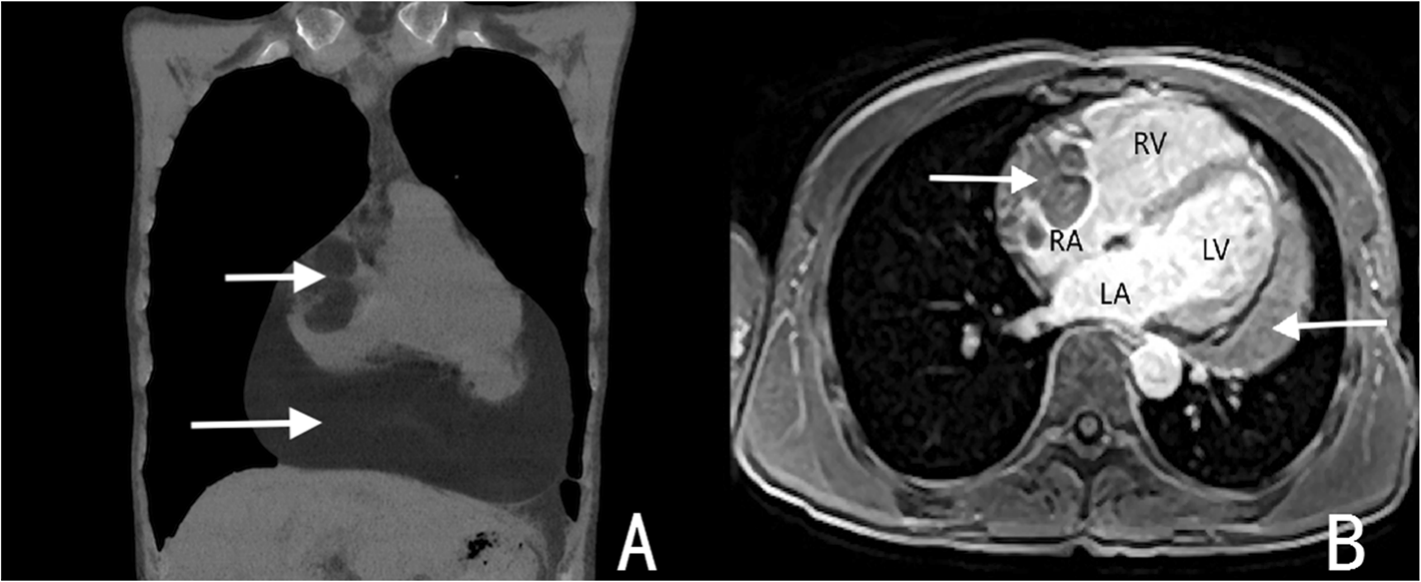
Magnetic resonance imaging examination. a In the coronal section, shows the pericardium and atrial mass (arrow) b In the long axis four chamber view, shows the pericardium and atrial mass (arrow)
